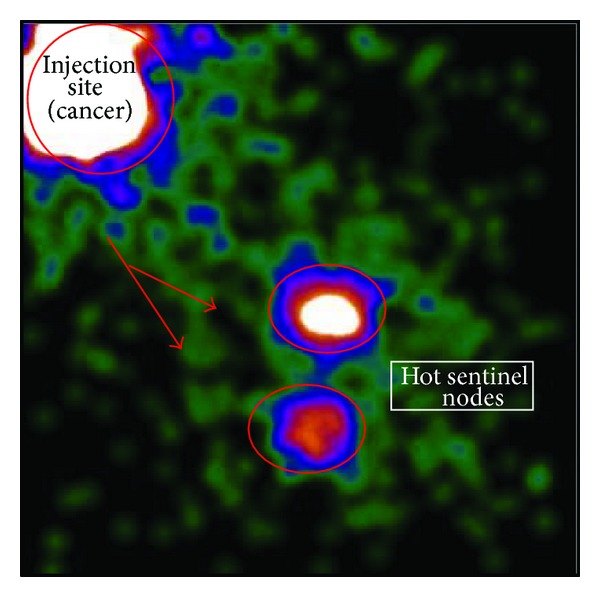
Intraoperative lymphoscintigraphy of patient with nonpalpable left breast cancer 3 hours after peritumoral injection of radiotracer (^99m^TC-labelled human albumin microcolloid). Anterior view shows 2 axillary sentinel nodes.
